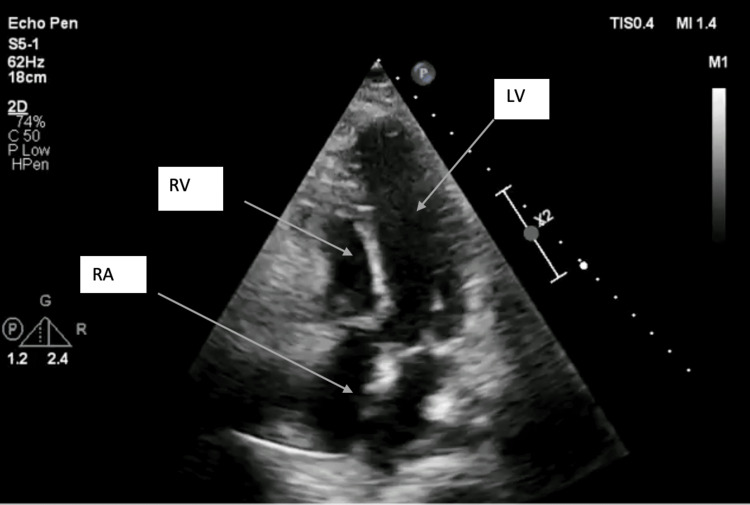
Post-procedure TTE showing successful cone repair LV, left ventricle; RA, right atrium; RV, right ventricle; TTE, transthoracic echocardiogram
